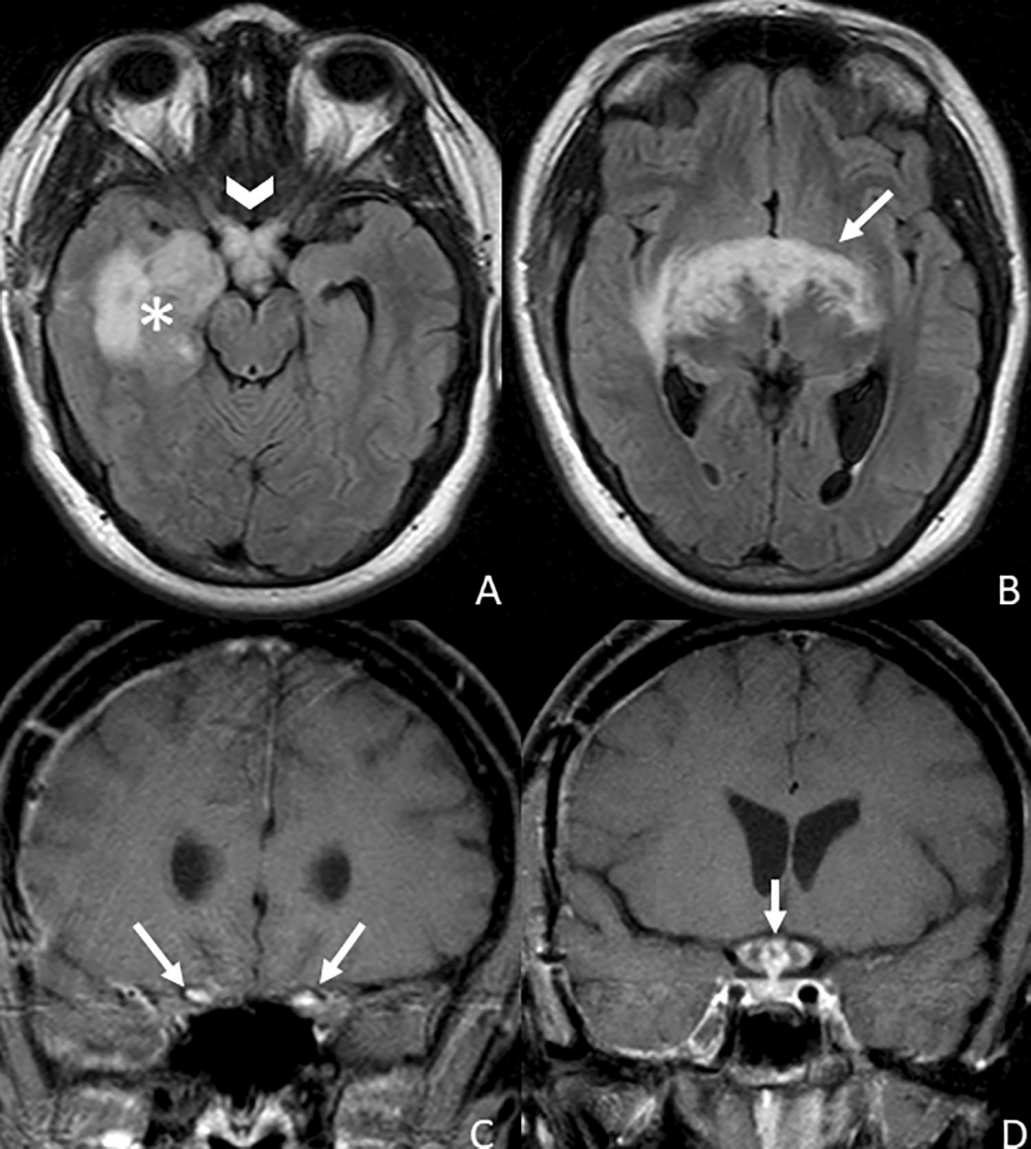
Axial FLAIR images (a, b) show marked hyperintensity of the region of the optic chiasm (arrowhead), involving the optic tracts (arrows) and extending into the right temporal lobe (asterisk) in a patient with neurosarcoidosis. This finding is correlated with a pathological bilateral thickened enhancement of the intracranial segment of the optic nerves (c, arrows) and of the optic chiasm (d, arrow)
